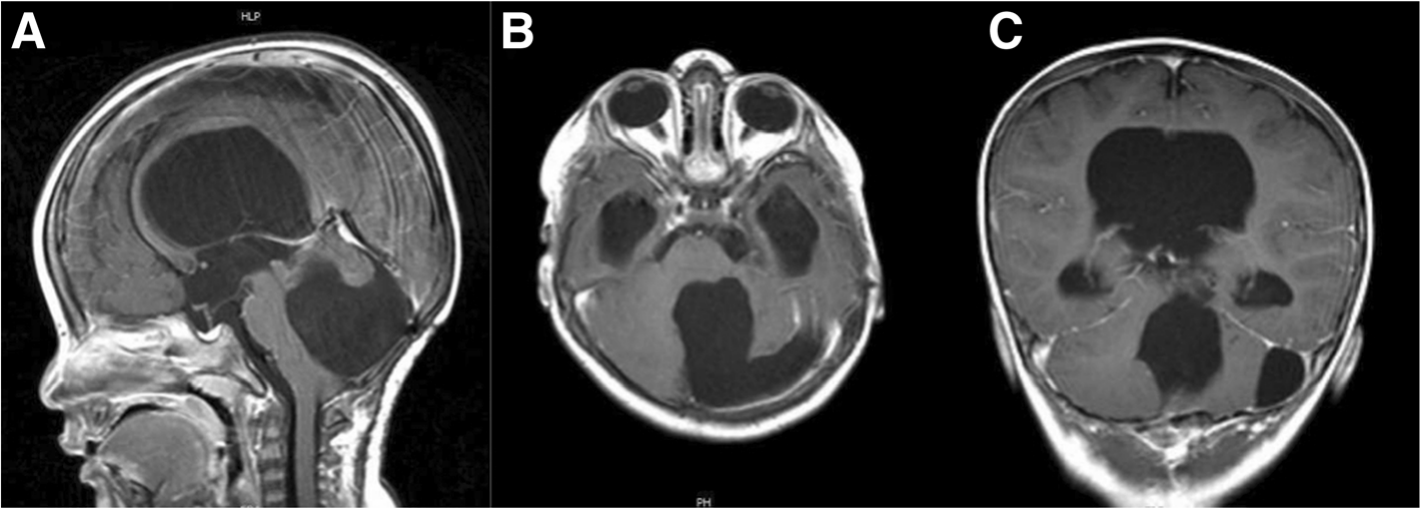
Follow-up at 12-month T1-weighted a sagittal, b axial, and c coronal MRI scans demonstrating no tumor recurrence
